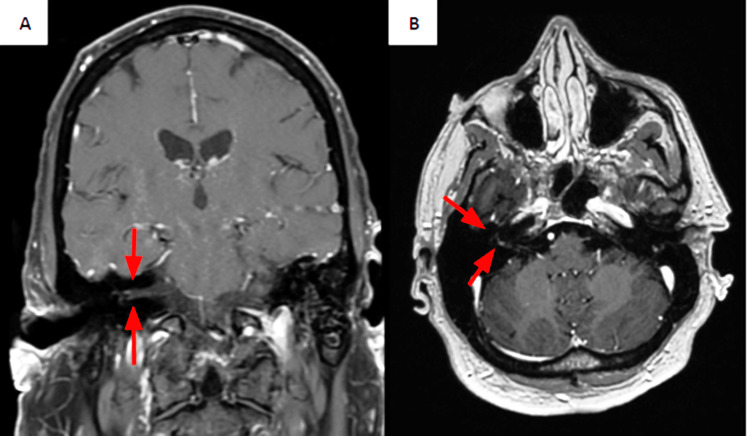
MRI of the head showing focal enhancement in the lateral right internal auditory canal (arrows). (A) T1-weighted coronal view. (B) T1-weighted axial view
